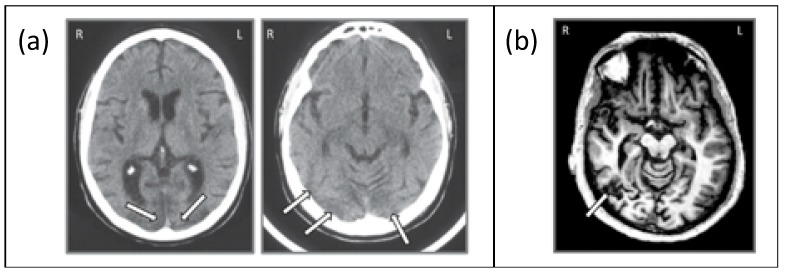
(a) Axial slice from the CT scan of JW showing bilateral posterior damage to parts of V1 and V2 and (b) axial slice from the MRI scan of SM showing lateralized damage to the right inferior temporal lobe. Adapted from Freud et al. [8], with permission from Oxford University Press, 2018.
